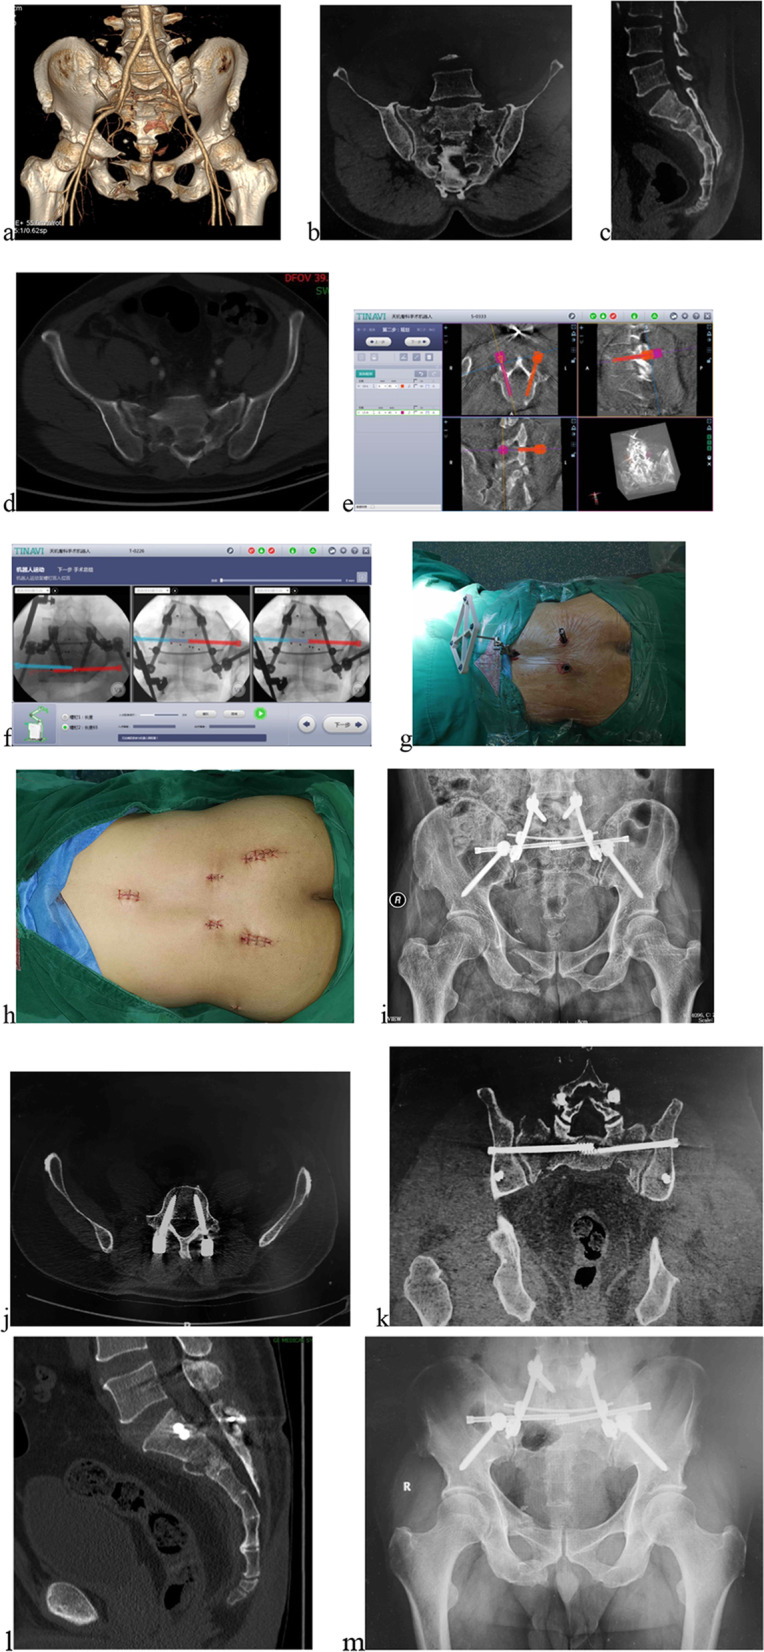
Male, 46 years old, a fall injury. a–d 3D CT reconstruction, coronal, sagittal and axial views after admission showing a type “H” sacral fracture associated with pubic superior and inferior rami fractures. e, f Planning of trajectories on bilateral L5 pedicle screws and bilateral S1 iliosacral screws. g Percutaneous pedicle screw implantation. h All sutured incisions. i Postoperative radiograph revealing bilateral triangular osteosynthesis for sacral fractures. j–l Postoperative CT views showing screws with good position. m Anteroposterior pelvic X-ray at one year after surgery demonstrating fracture healing
